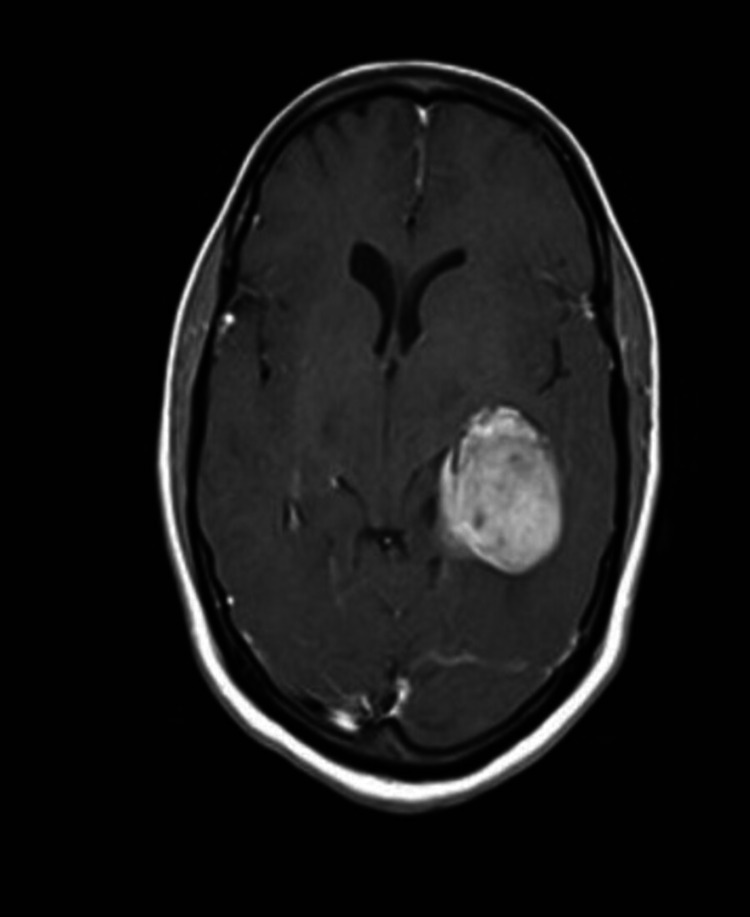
Preoperative MRI brain contrast - A
